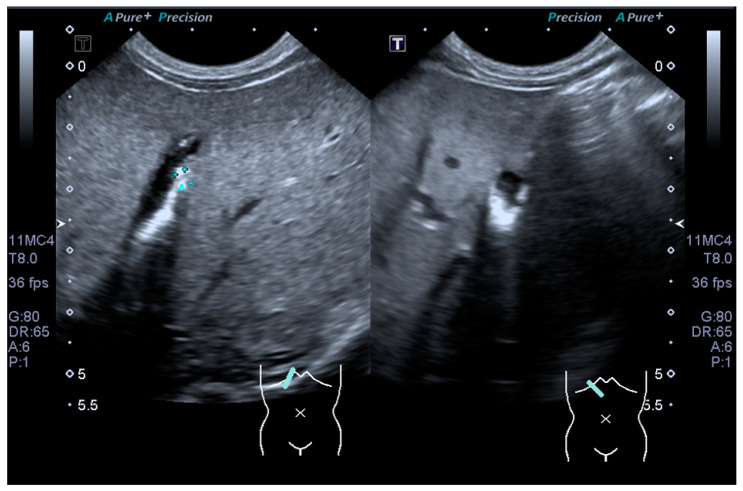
Ultrasound picture showing multiple echogenic foci within the gallbladder with posterior shadowing suggestive of sludge.
